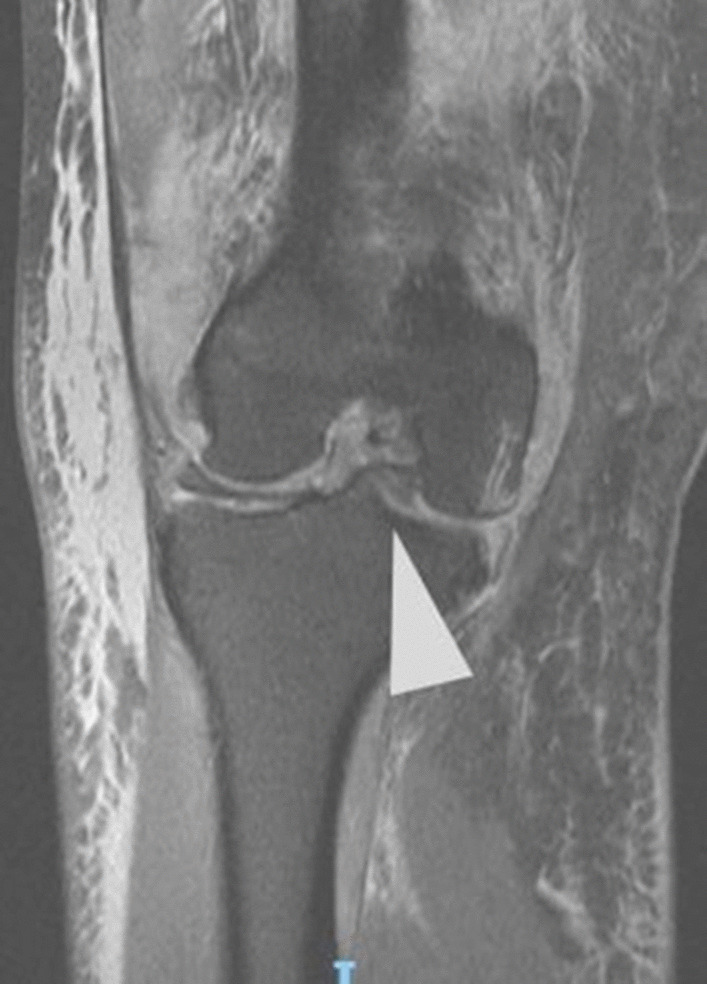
Coronal magnetic resonance imaging demonstrating bucket handle tear of medial meniscus with segment flipped into intercondylar notch, indicated by arrowhead
